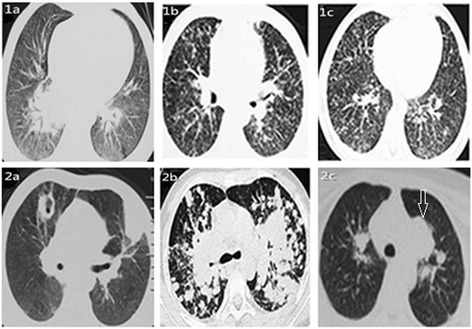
Chest HRCT scans showing the presence of diffuse nodular opacities and slight ground-glass in bilateral inferior fields (1a; on admission) and consolidation in left upper lobe and cavity in right upper lobe (2a; after treatment for three weeks) in case 1; bilaterally diffuse ill-defined centrilobular nodules and slight ground-glass (1b; on admission) and multi-nodules fused into pieces more in upper lung (2b; after treatment for 3 weeks) in case 2; and bilaterally diffuse ill-defined centrilobular nodules and slight ground-glass (1c; on admission), consolidation with halo (arrow) in left upper lobe (2c; after treatment for one month) in case 3
